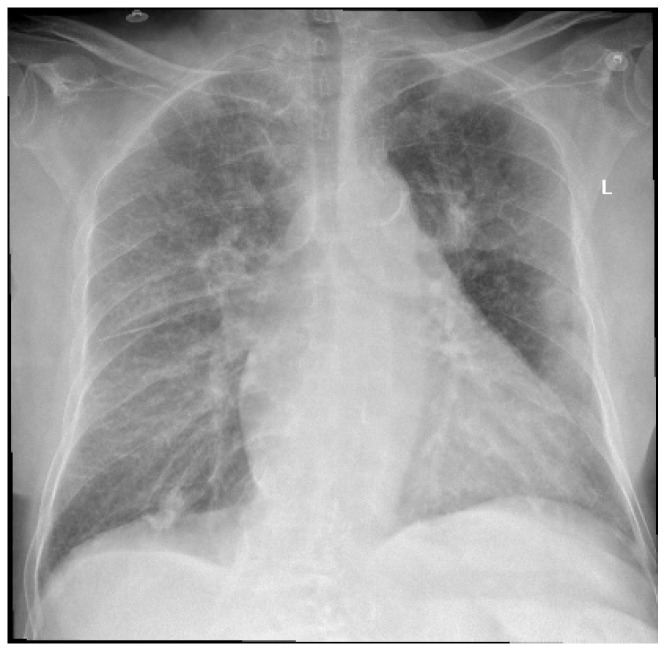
Chest X-ray at admission—describing small left pleural effusion; bilateral diffuse reticule-micronodular interstitial lung pattern; questionable opacities at the level of the posterior left VI costal arch and right intercostal XI space; pulmonary vascular congestion; global enlarged heart; atherosclerotic aorta. L—left side.
